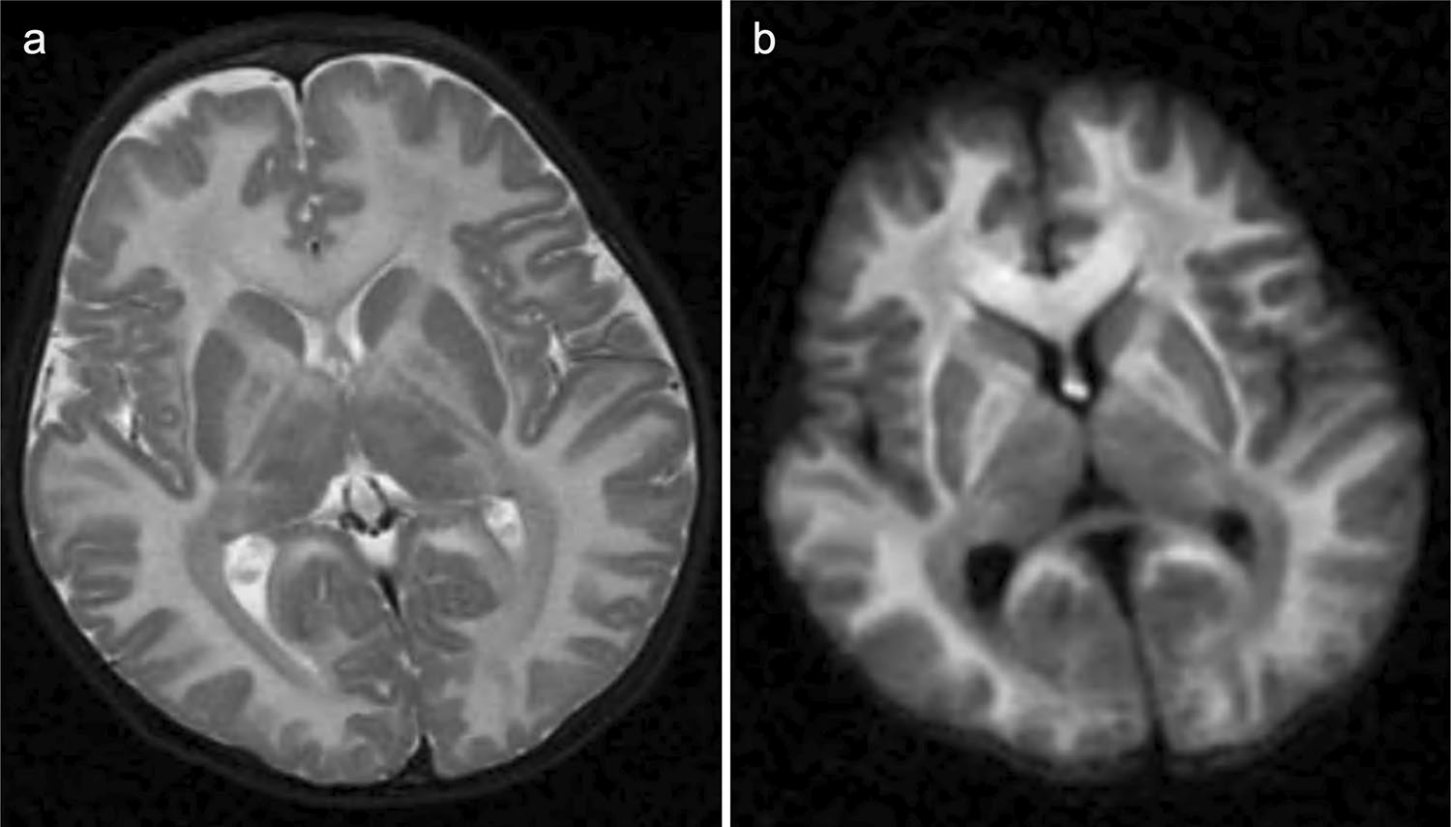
A 2-year-old, male patient with cystathionine β-synthase deficiency (CBSD). a, b T2-weighted imaging (a) and DWI (b) demonstrated diffuse T2 hyperintensity and restricted diffusion of the cerebral white matter, corpus callosum, anterior limb of the internal capsules, fornices, and globi pallidi
